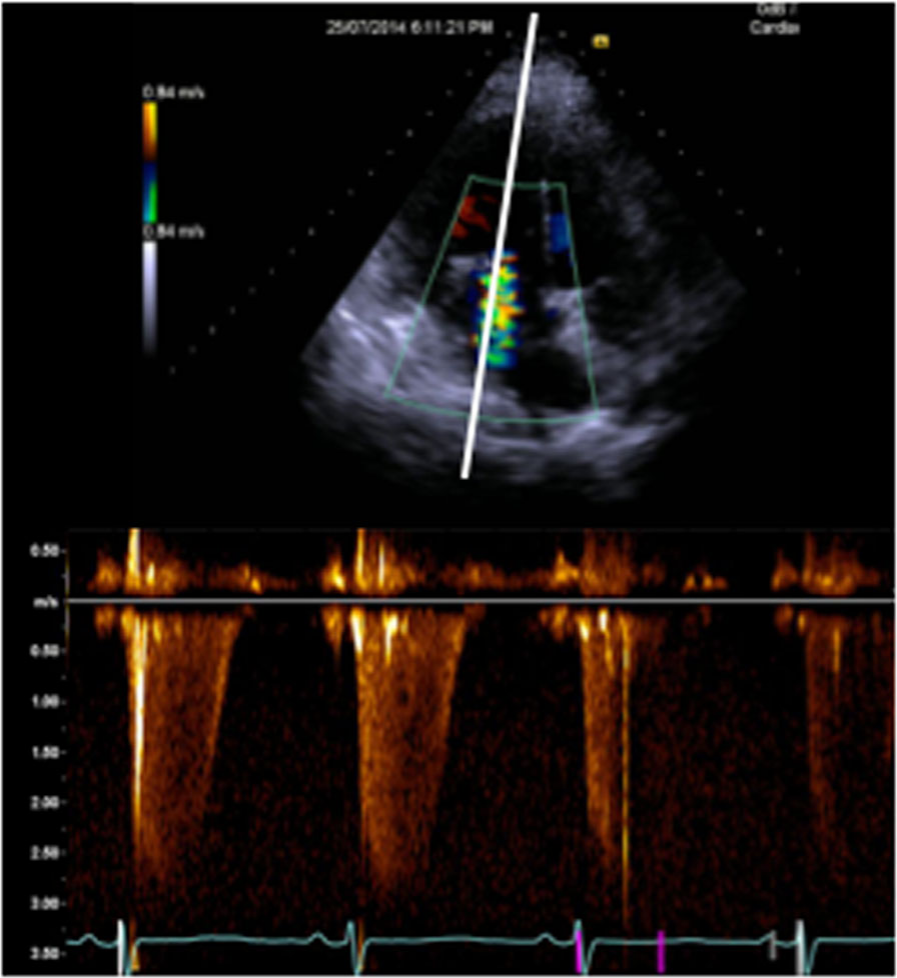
Utility of using non-standard imaging windows. Apical right ventricle-centric views (probe positioned more medially than standard apical view): enables better Doppler angle for tricuspid regurgitation assessmentWith dilated cardiomyopathy, blood flow from the left atrium into the LV can become increasingly eccentric and directed towards the lateral wall. Using colour Doppler to view direction of blood flow into the LV and placing the probe more laterally can help ensure an accurate Doppler angle.
